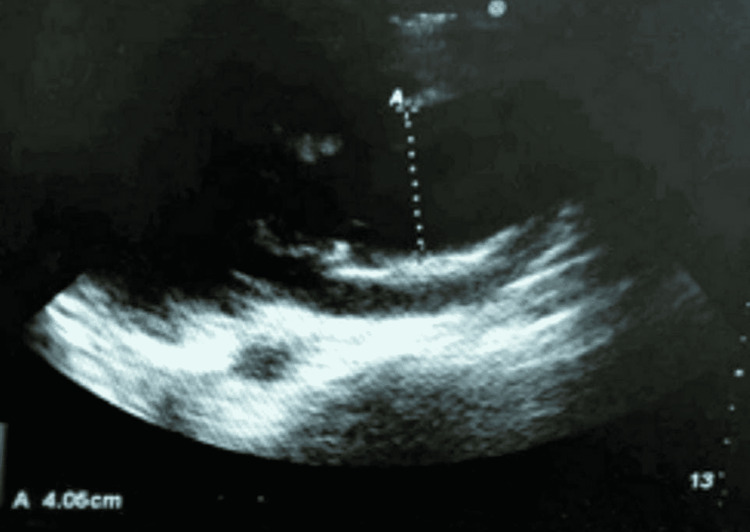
Aortic root diameter of 4.06 cm (point-of-care ultrasound)
